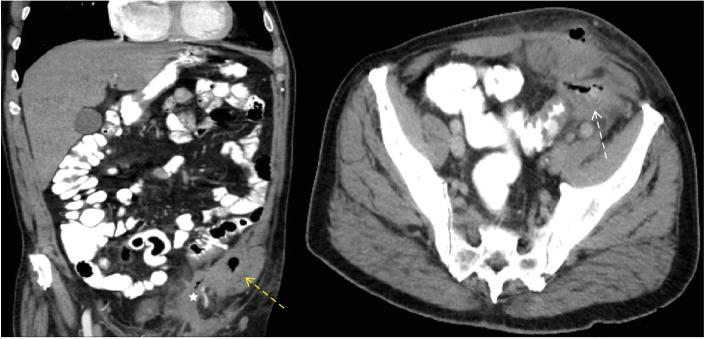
Computed tomography of the abdomen; the picture highlights an inflamed 4.6 × 9.5 cm sized conglomerate adjacent to the abdominal wall and the left inguinal canal (yellow and white dashed arrow). The prior implanted mesh seemed to be partially dislocated to the abdominal cavity (white star).
